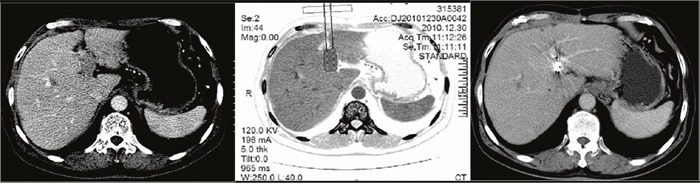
A 65-year-old man with hepatocellular carcinoma complicated with portal vein tumor thrombus in the left branch Enhanced computed tomography showed the thrombus originated from the left branch of the portal vein. The image prior to iodine seed implantation showed the spatial distribution of seeds according to the treatment planning system. Computed tomography demonstrated that the portal vein tumor thrombus decreased in size and that the portal vein was recanalized two months after seed implantation.
